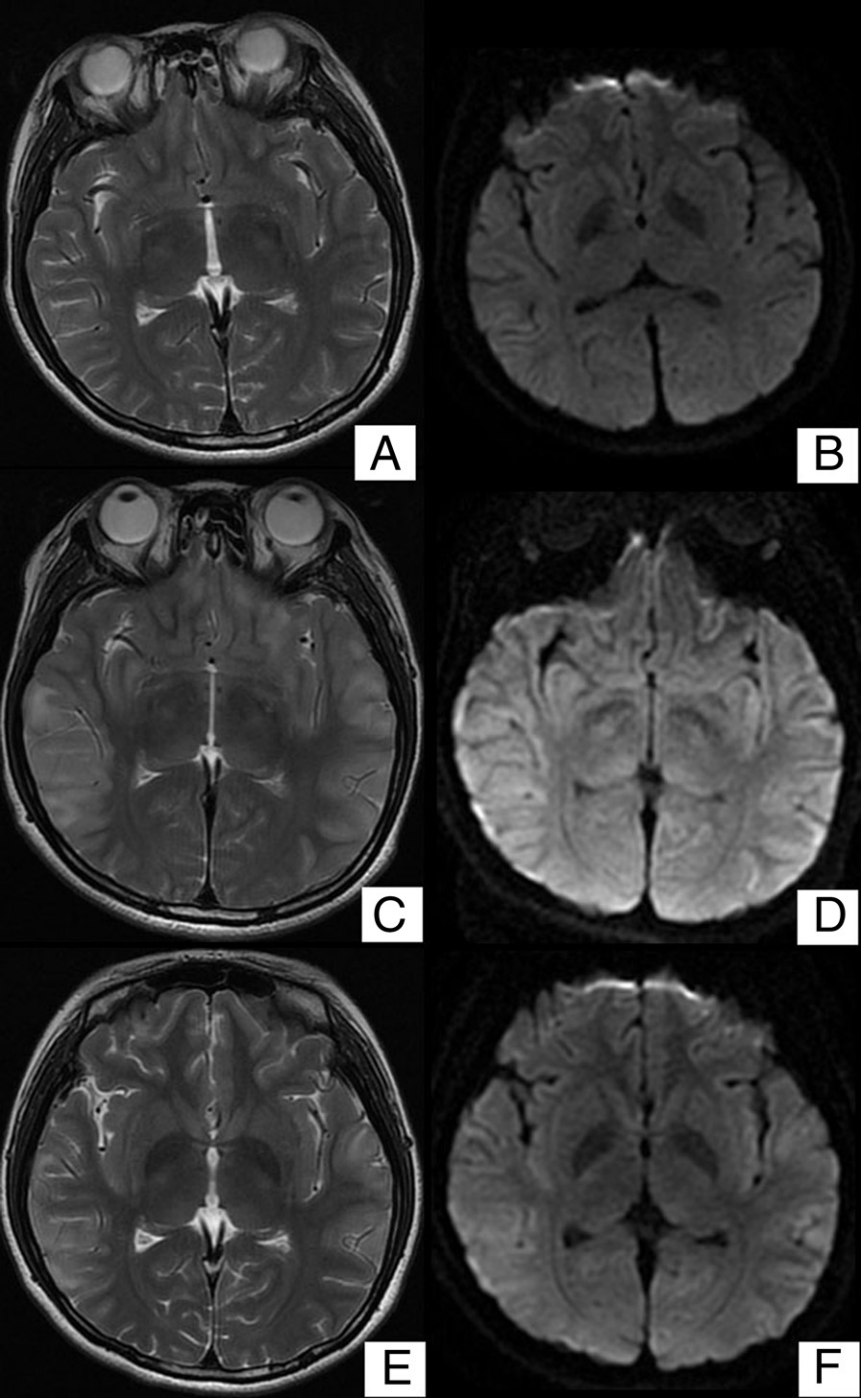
MRI of a 19 year-old SLE patient who presented with stupor. T2-weighted image (A) and Diffusion-weighted image (B) show normal. After 2 weeks repeat MRI, T2-weighted image (C) shows high signal lesions in bilaterally temporooccipital white matter. DWI (D) shows lesion isointensity. Follow-up T2-weighted image (C) obtained 2 week after (E) reveals almost resolution of high signal area in bilaterally lesion region. DWI (F) reveals lesion isointensity.
